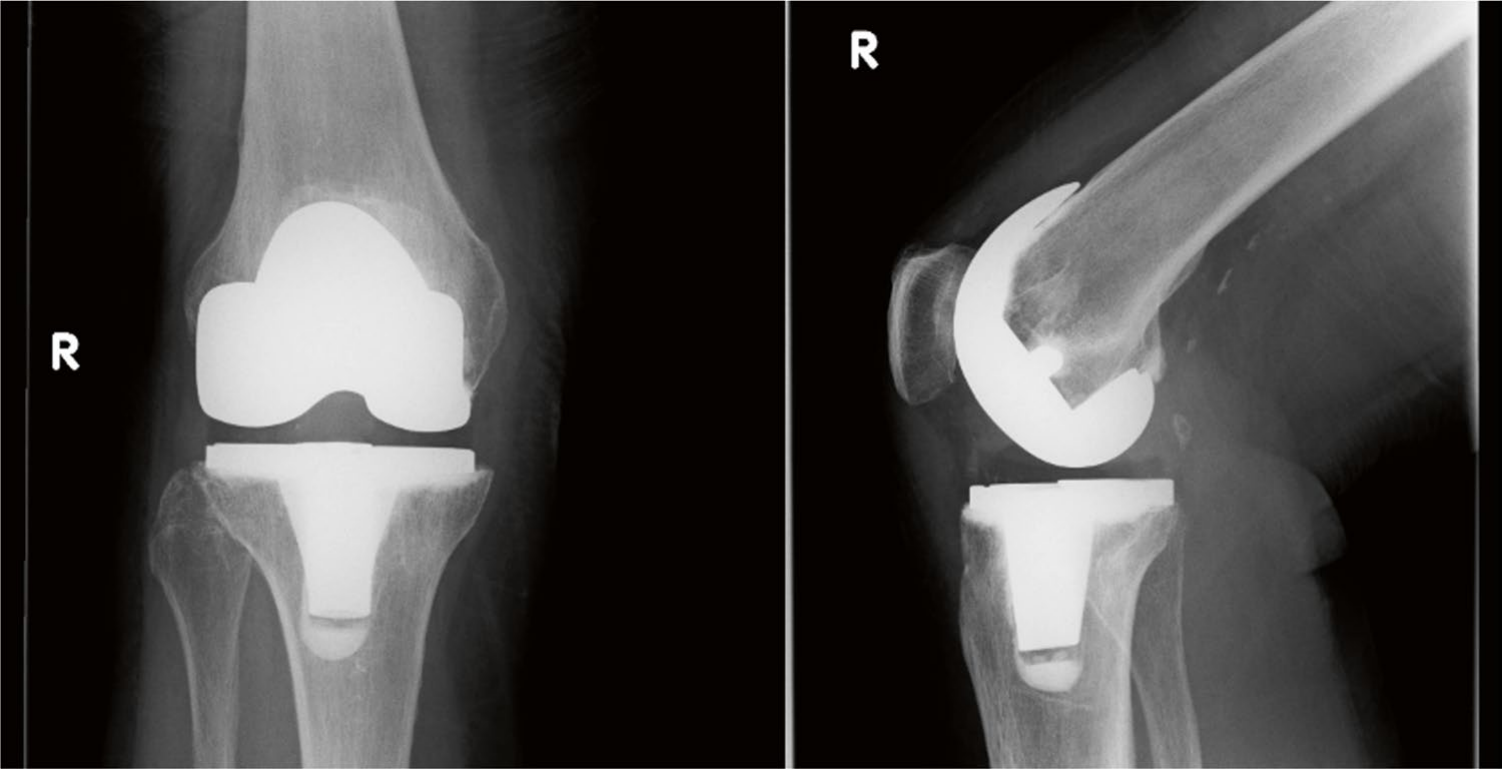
Anteroposterior (AP) and lateral radiographs of an augmentable prosthesis. This component is identifiable by the presence of a radiolucent polyethylene peg at the tip of the keel on the tibial baseplate
